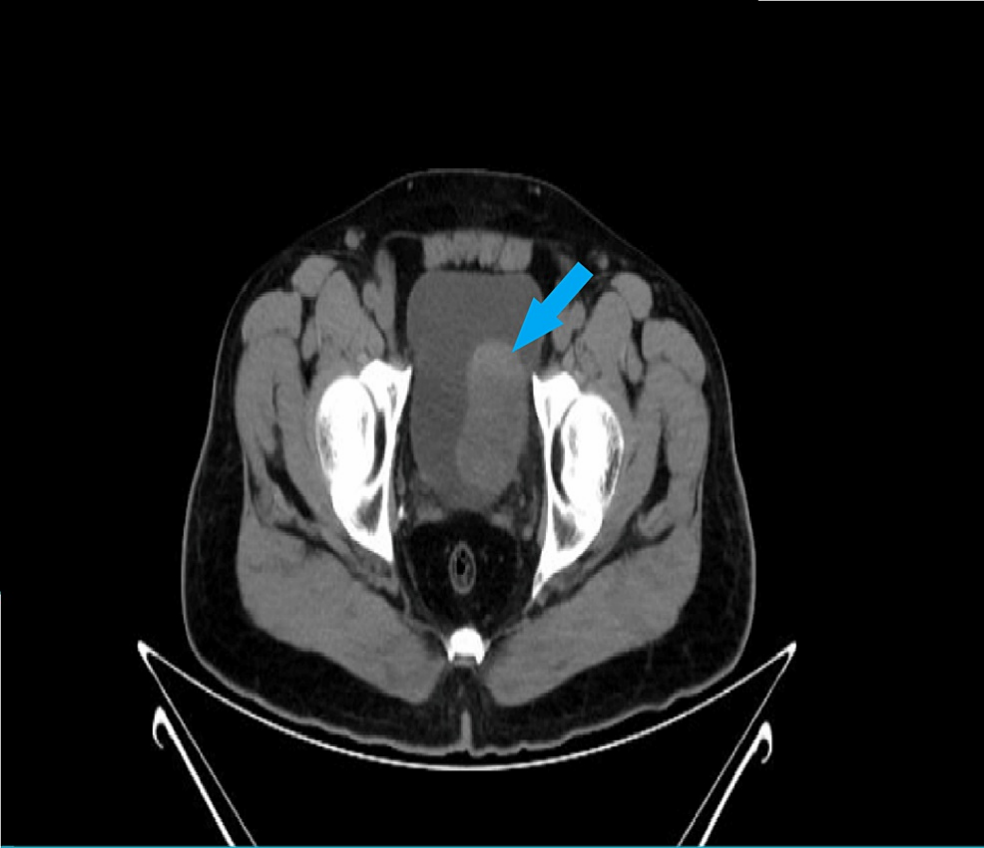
CT urography showing primary malignant neoplastic lesion of the urinary bladder. A large lobulated endophytic irregularly outlined heterogenous lesion of predominantly soft tissue attenuation protruding into the bladder lumen (arrow).
